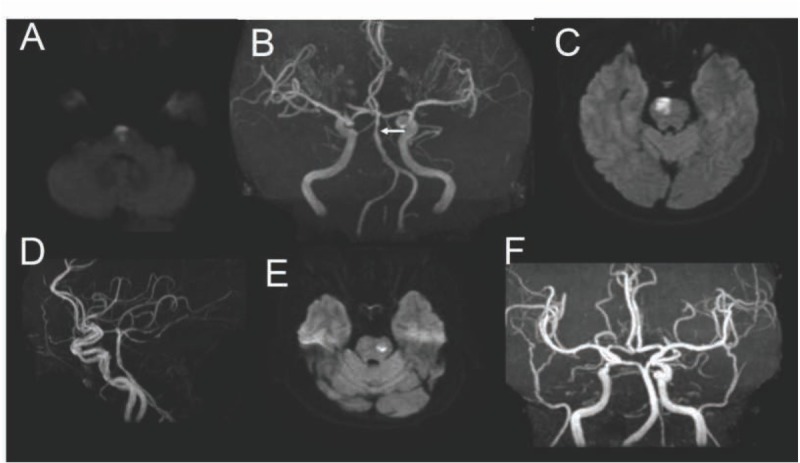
Paramedian pontine infarct (PPI) and lacunar pontine infarct (LPI) in brain imaging of DWI and MRA image. (A) Axial DWI showing a PPI. (B) MRA showing stenosis of the basilar artery of the same patient as A. (C) Axial DWI showing a PPI. (D) The basilar artery of the same patient as C is normal; (E) Axial DWI showing an LPI. (F) The basilar artery of the same patient as E is normal.
